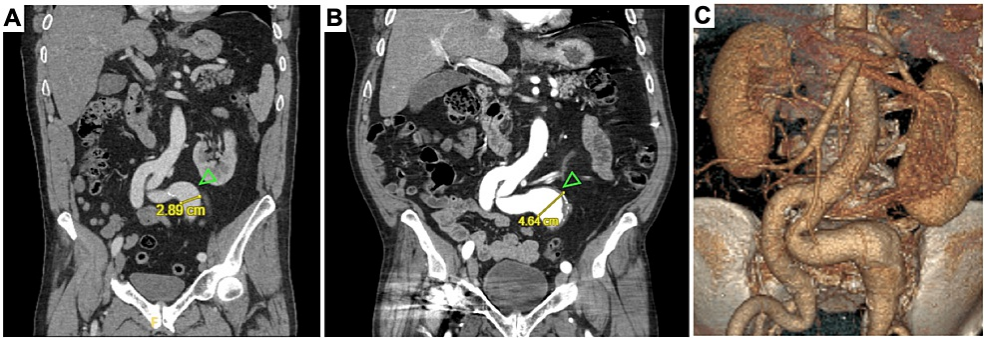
Left iliac artery aneurysm. CT angiograms of the abdomen and pelvis showing the left common iliac artery aneurysm (green arrowheads) on (A) initial presentation, measuring 2.9 cm, and (B, C) 10 years later, measuring 4.6 cm. Also shown is marked tortuosity of the left common iliac artery.
